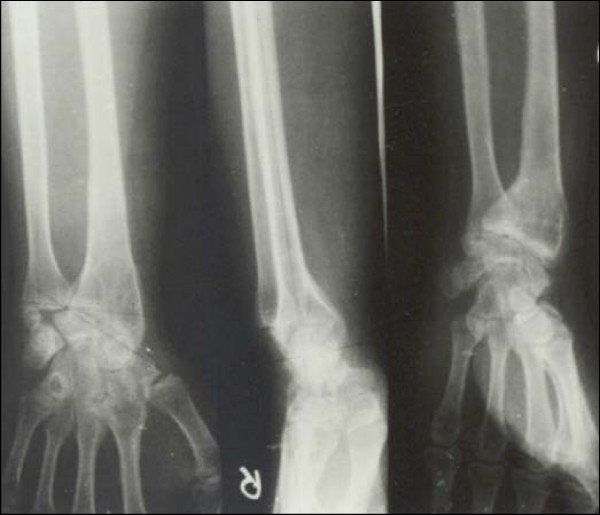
Case 3 Radiograph at 7 year followup Anteroposetrior and Lateral view.
